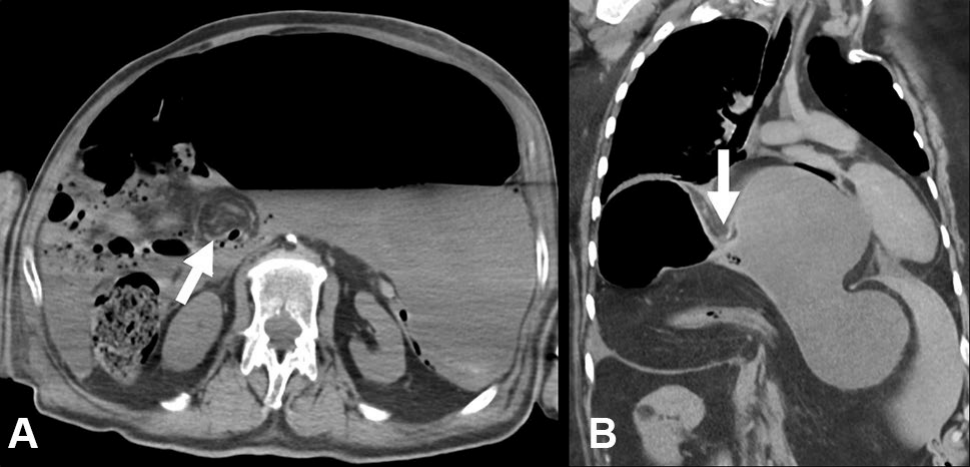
Post-mortem CT findings in gastric volvulus: case 1 (A) and case 2 (B). Axial reconstruction of the stomach showing massive gas and fluid-filled distension with a lesser omental “whorl” sign (arrow), indicative of organoaxial volvulus. Coronal multiplanar reconstruction showing a distended fluid-filled stomach with “waisting” in the mid gastric body (arrow), indicating the site of organoaxial volvulus within a hiatus hernia
